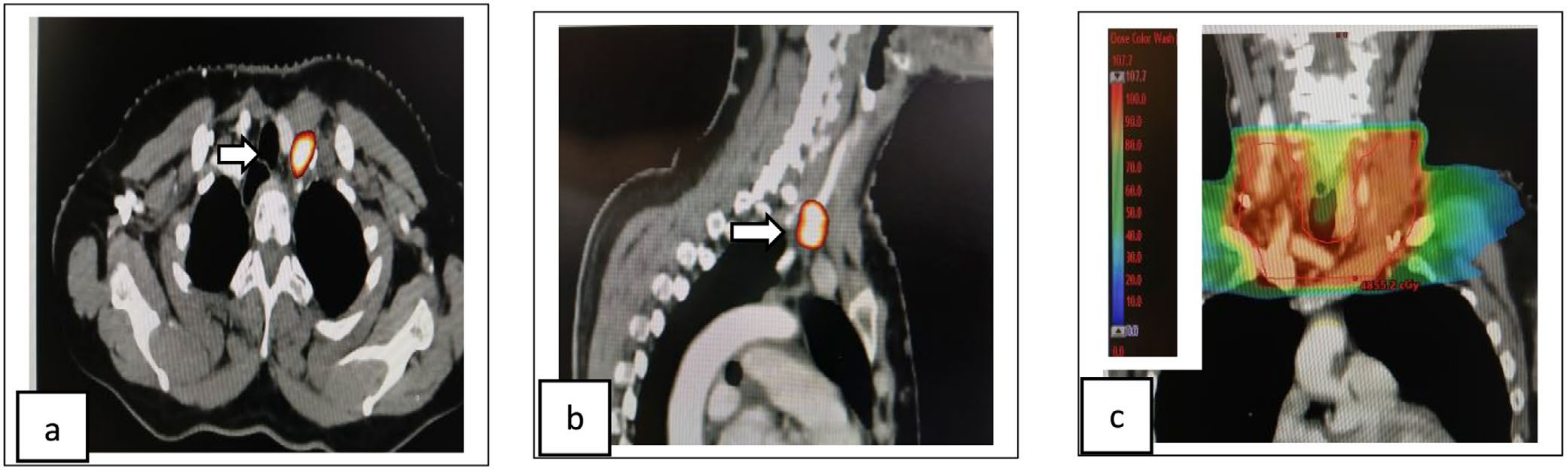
First recurrence in left SCF. (a, b) White arrow in axial and sagittal sections of CT superimposed with PET shows left SCF nodal recurrence. (c) Coronal CT section shows dose color wash of SCF region treatment volume.
